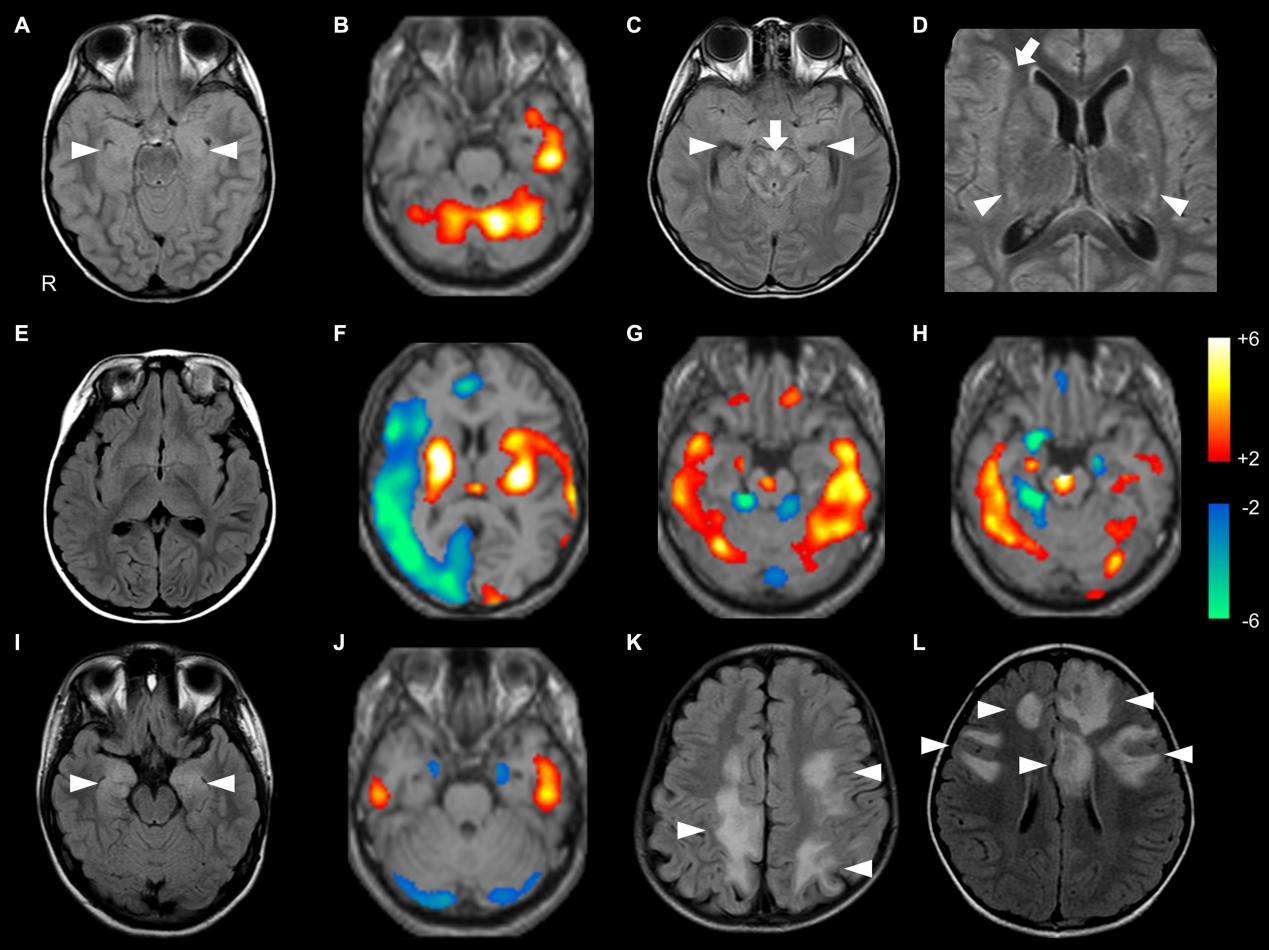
Brain MRI and SPECT findings of probable Ab-negative pediatric autoimmune encephalitis (A–H) and major antibody-positive autoimmune encephalitis (NMDARE: I,J; MOGAD: K,L). In 99mTc-ECD-SPECT images, a regional Z-score of > +2.0 or < −2.0 was considered significant (B,F–H,J). (A,B) The same patient. (A) FLAIR showed high signal intensities in the bilateral medial temporal lobes (arrowheads). (B) ECD-SPECT showed increased blood flow in the left temporal lobe and bilateral cerebellum. (C,D) The same patient. FLAIR showed high signal intensities in the bilateral medial temporal (C, arrowheads), brainstem (C, arrow), basal ganglia (D, arrowheads), and right insula (D, arrow) with negative anti-glial fibrillary acidic protein antibodies. (E,F) The same patient. (E) MRI showed no obvious abnormalities. (F) ECD-SPECT showed diffusely decreased blood flow in the right frontal, lateral temporal, insular, and occipital regions and increased blood flow in the bilateral basal ganglia, left insular, lateral temporal, and occipital regions. (G) Even in a patient without MRI abnormalities, ECD-SPECT showed increased blood flow in the bilateral lateral temporal lobes, cerebellum, frontal lobe, right medial temporal lobe, and brainstem. (H) In another patient, ECD-SPECT showed increased blood flow in the bilateral lateral temporal lobes, cerebellum, and brainstem; increased or decreased blood flow in the right medial temporal lobe; and decreased blood flow in the left medial temporal lobe. (I) FLAIR demonstrated high signal intensities in the bilateral medial temporal regions in a patient with NMDARE (arrowheads). (J) ECD-SPECT exhibited increased blood flow in the bilateral lateral temporal lobes and decreased blood flow in the bilateral medial temporal lobes and cerebellum in an NMDARE patient without MRI abnormalities. (K,L) FLAIR showed multiple high signal intensities in the bilateral hemisphere in patients with MOGAD (arrowheads). ECD-SPECT, ethyl cysteinate dimer-single-photon emission computed tomography; FLAIR, fluid-attenuated inversion recovery; MOGAD, myelin oligodendrocyte glycoprotein-associated disease; NMDARE, anti-N-methyl-D-aspartate receptor encephalitis; Probable Ab-negative AE, probable antibody-negative pediatric autoimmune encephalitis.
